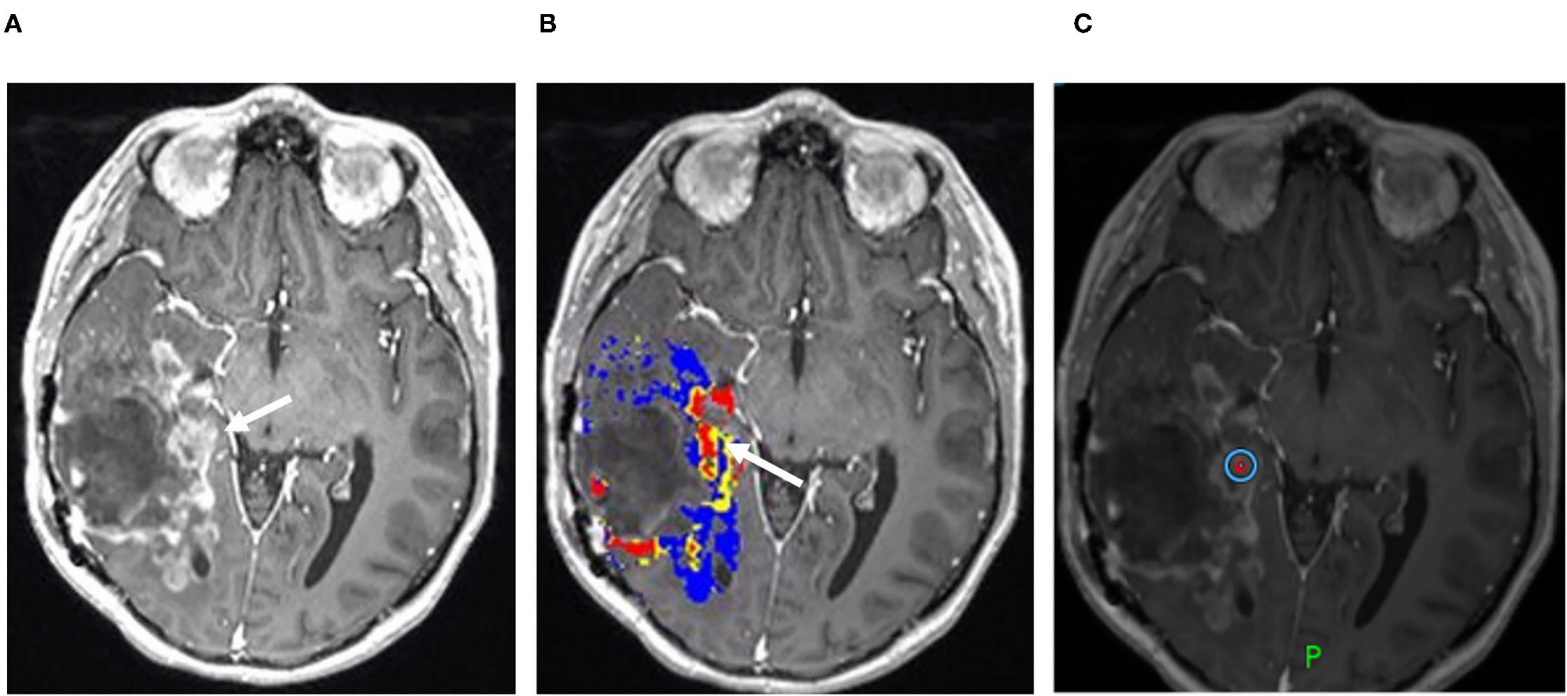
Recurrent tumor on DSC-MRI: A 27-year-old man with right temporoparietal GBM with prior surgical resection with carmustine wafer placement and adjuvant radiation/temozolomide. Five months after surgical resection, the patient experienced clinical deterioration with signs of increased intracranial pressure with clinical concern for tumor progression vs. pseudoprogression. His symptoms did improve with steroid therapy. (A) Axial postcontrast T1 weighted image shows a large area of nodular and ring-like enhancement along the medial margin of the resection cavity (arrow). (B) The DSC FTB map derived from rCBV thresholds demonstrates high FTB centrally in the area of nodular enhancement consistent with the tumor. (C) The patient underwent surgical debulking, and the stereotactic biopsy at the site of the elevated rCBV (circular marker) was positive for 80% recurrent viable tumor.
